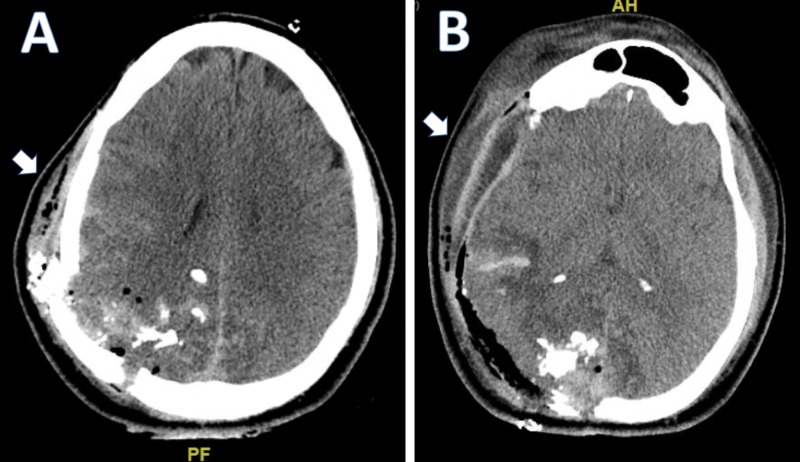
Initial frontotemporoparietal craniectomy pre-op (A) and post-op (B) CT imaging CT, computed tomography
